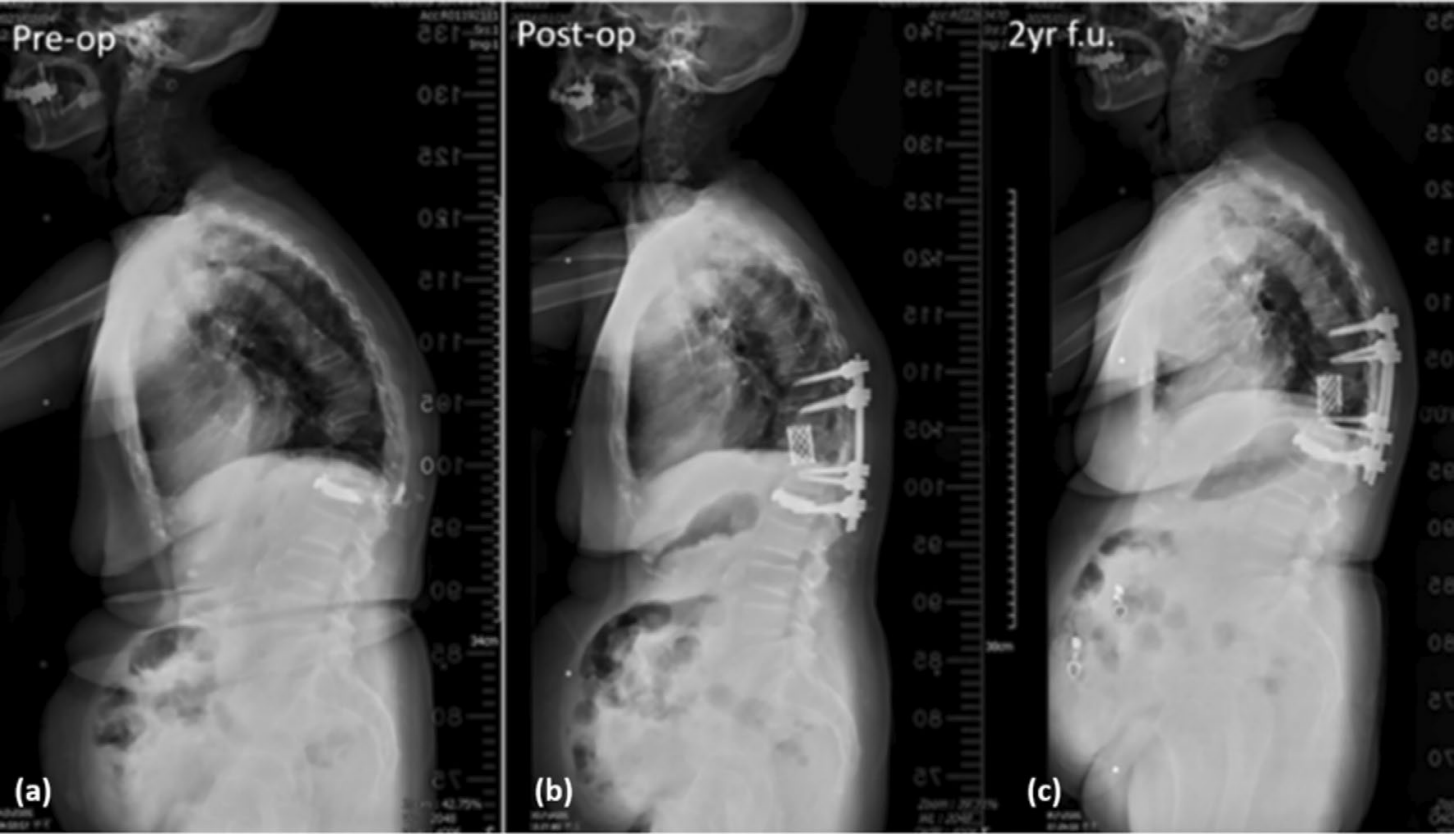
(a) An 81‐year‐old female presented with thoracolumbar kyphosis secondary to OVF and decompensated sagittal imbalance (TLK: 39°; SK: 37.7°; OH: 26.8 mm). (b) She underwent T11 LCR‐A combined with PCO and PSF (T9–L1) under navigation in the lateral decubitus position. The procedure achieved significant sagittal correction (TLK: 22.8°; SK: 4°; OH: 33.7 mm), with an estimated blood loss of 200 mL and operative duration of 316 min. No drainage was required, and the patient was able to ambulate on postoperative day one. (c) Radiographic follow‐up at 2 years demonstrated well‐maintained correction (TLK: 24.5°; SK: 5°; OH: 32.5 mm).
